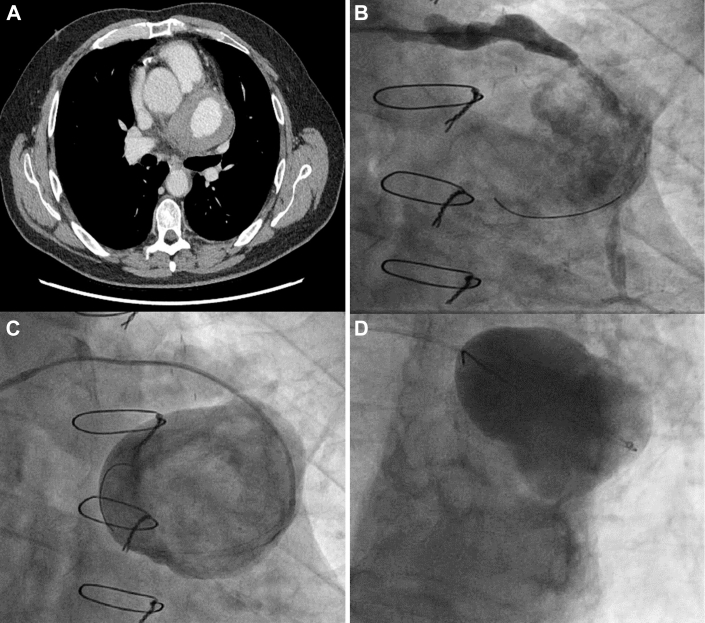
Findings on Initial Imaging Chest x-ray revealing prominence of the mediastinal contour and pulmonary artery. (A) Cardiac computed tomography showing a large pseudoaneurysm of the saphenous vein graft. (B to D) Coronary angiography showing different views of the large aneurysm.
